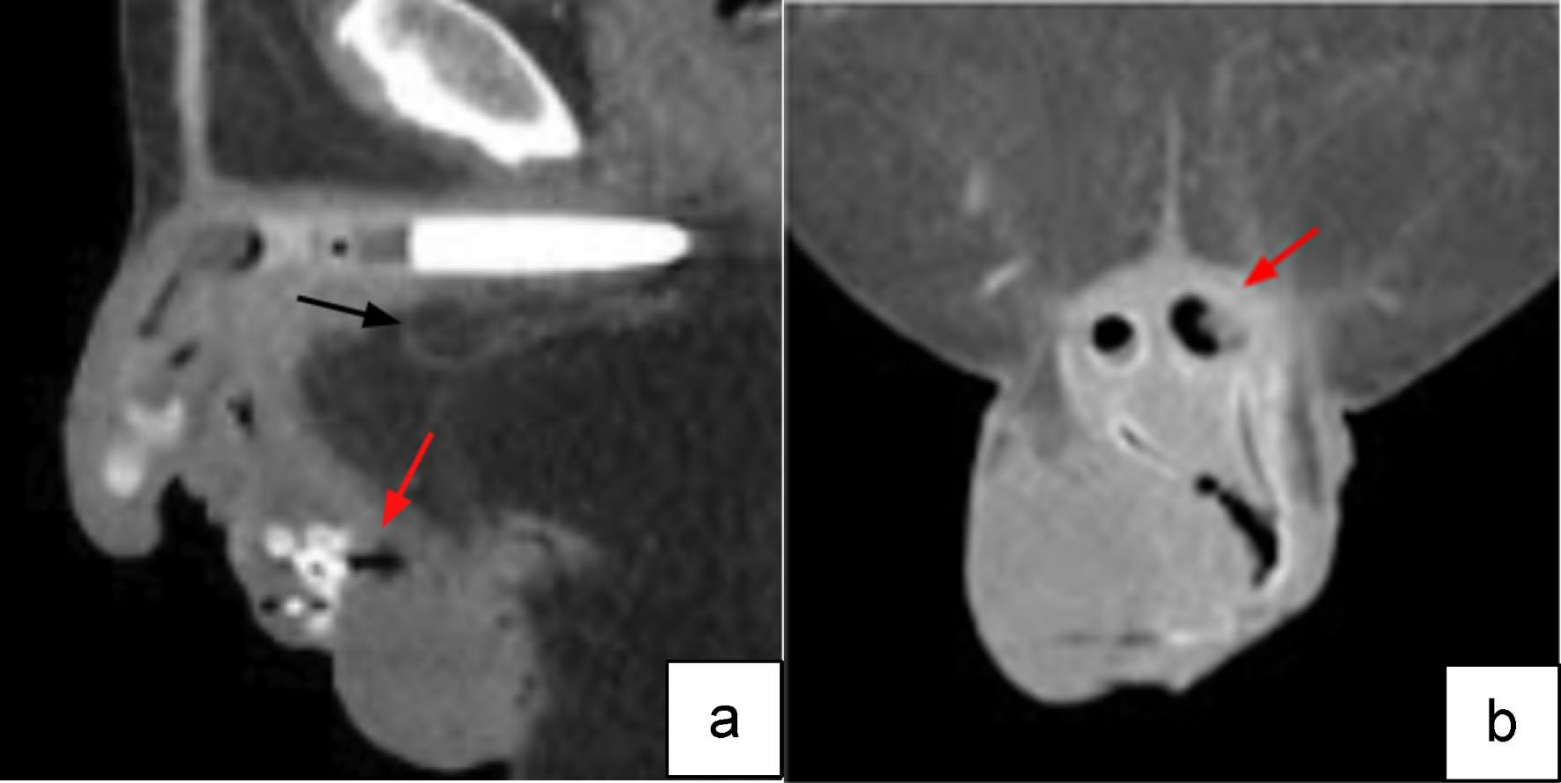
Sagittal (a) and coronal (b) CT images demonstrate gas (red arrows) within the scrotum and corpora cavernosa components of the PP with surrounding fat stranding (black arrows) compatible with infection
